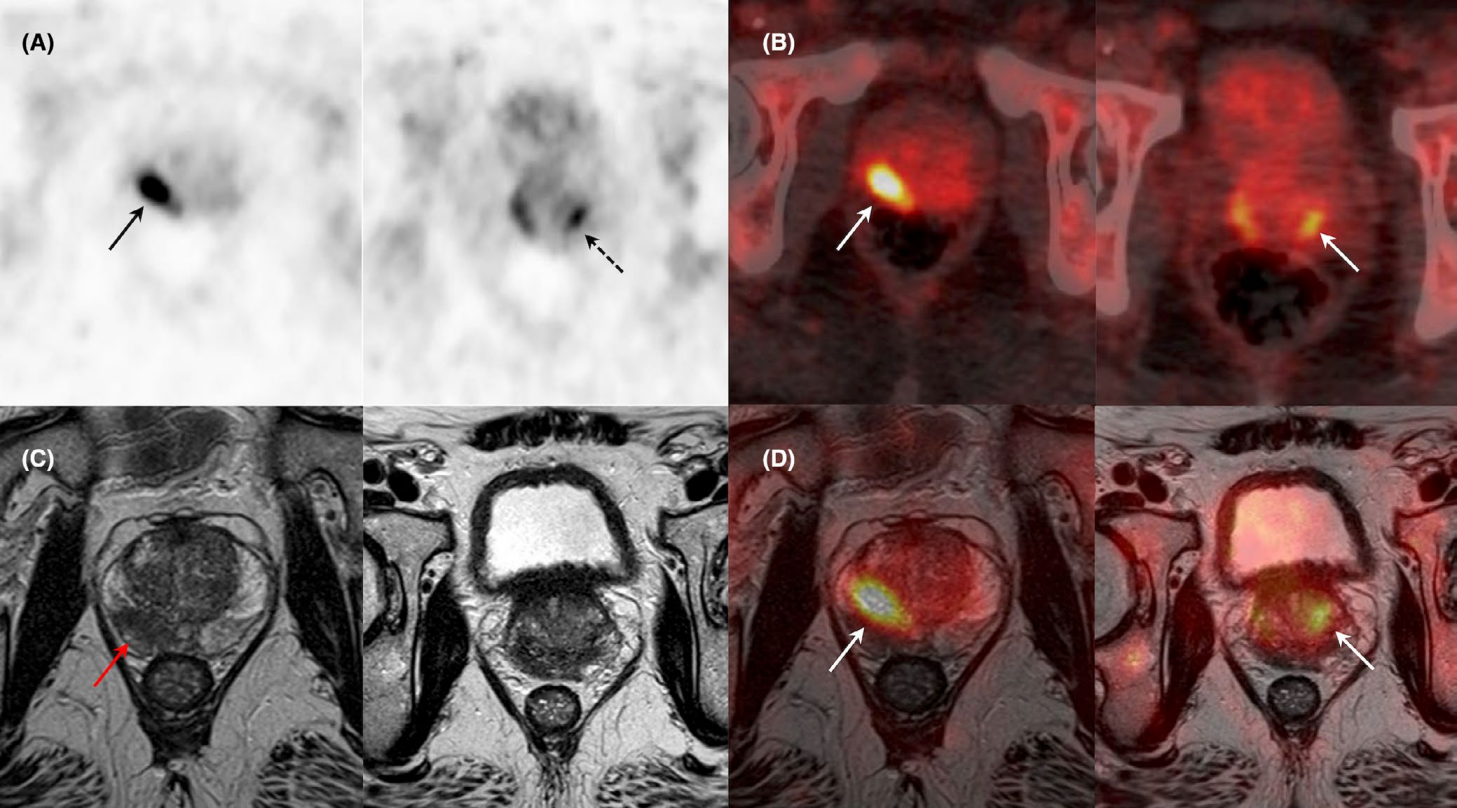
Results of [^18^F]PSMA1007 PET/CT and MRI imaging (2‐levels axial PET (A), PET/CT (B), T2‐weighted MRI (C), PET/MRI fused images (D)) in a patient with biological suspicion of prostate cancer (PSA: 9 ng/mL). Both PET and MRI showed a pathological lesion, involving the posterolateral and posteromedial right peripheral zone (19 × 13 × 8 mm, SUVmax 13, MRI PI‐RADS: 5). An additional [^18^F]PSMA1007 focal uptake (SUVmax 8.56) was revealed in the left transitional zone. Pathological examination after biopsy confirmed the presence of acinar adenocarcinoma (both lesions), Gleason score 7 (4 + 3).
